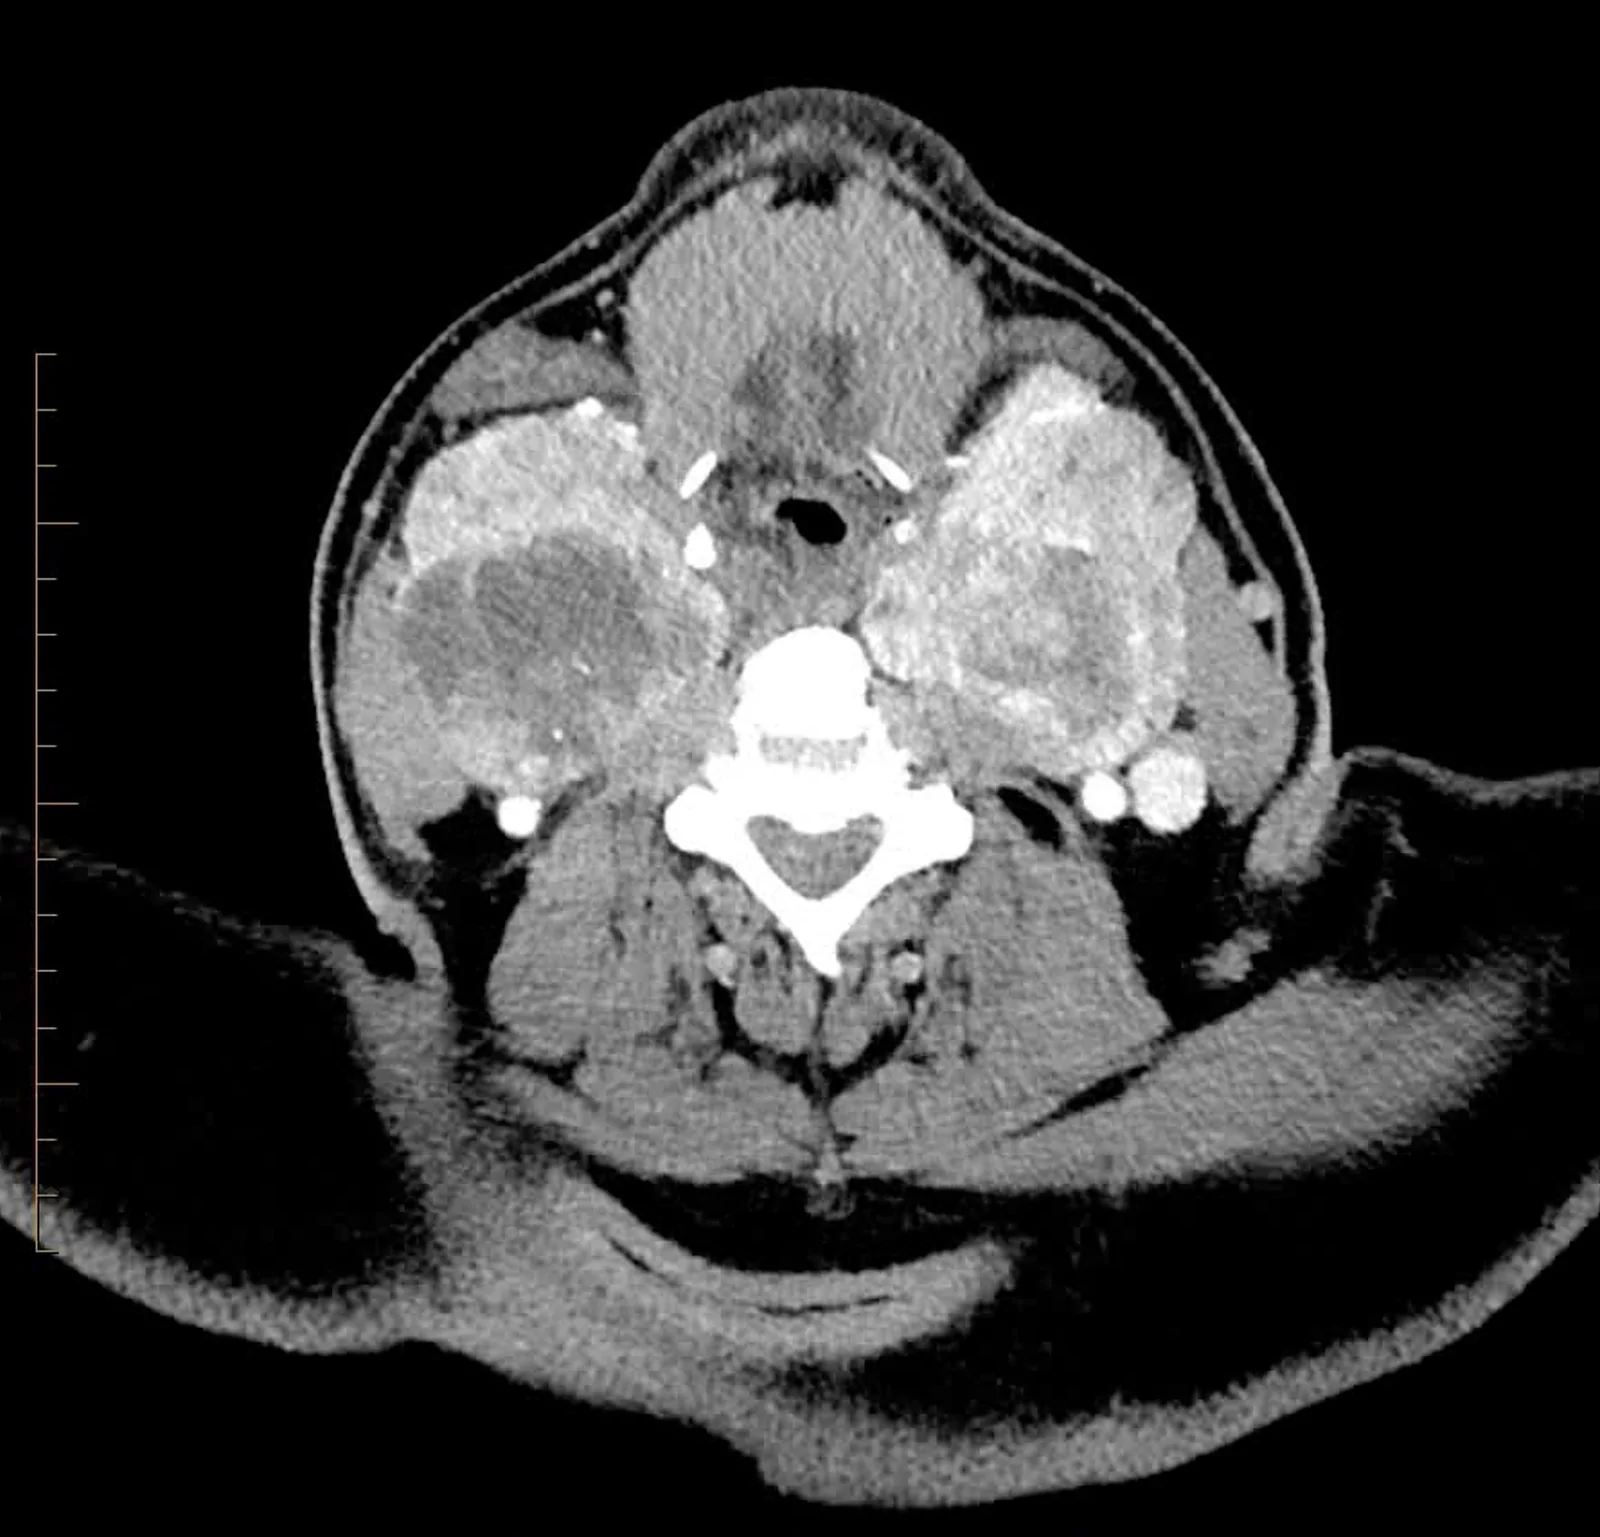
Computed tomography scan of the neck showed a diffusely enlarged thyroid gland, with the right lobe measuring 5.5 × 6.1 × 9.6 cm and the left lobe measuring 6.3 × 7.5 × 12.9 cm. Source: JC.
